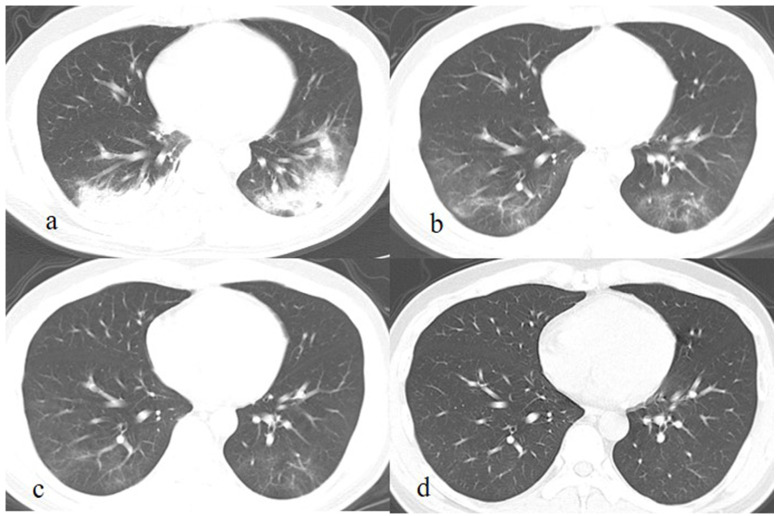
A patient exposed to COVID-19 presents with fever, cough, and fatigue for 7 days. The initial chest CT at the first day after admission shows patchy consolidations and GGO in the left and right lower lobes (a). Subsequently (7 and 13 days later), the lesions are continuously absorbed with decrease of extent and density, and fibrous strips are detected in lesions (b, c). On the latest CT scan (36 days later), the lesion is completely absorbed GGO (c).
